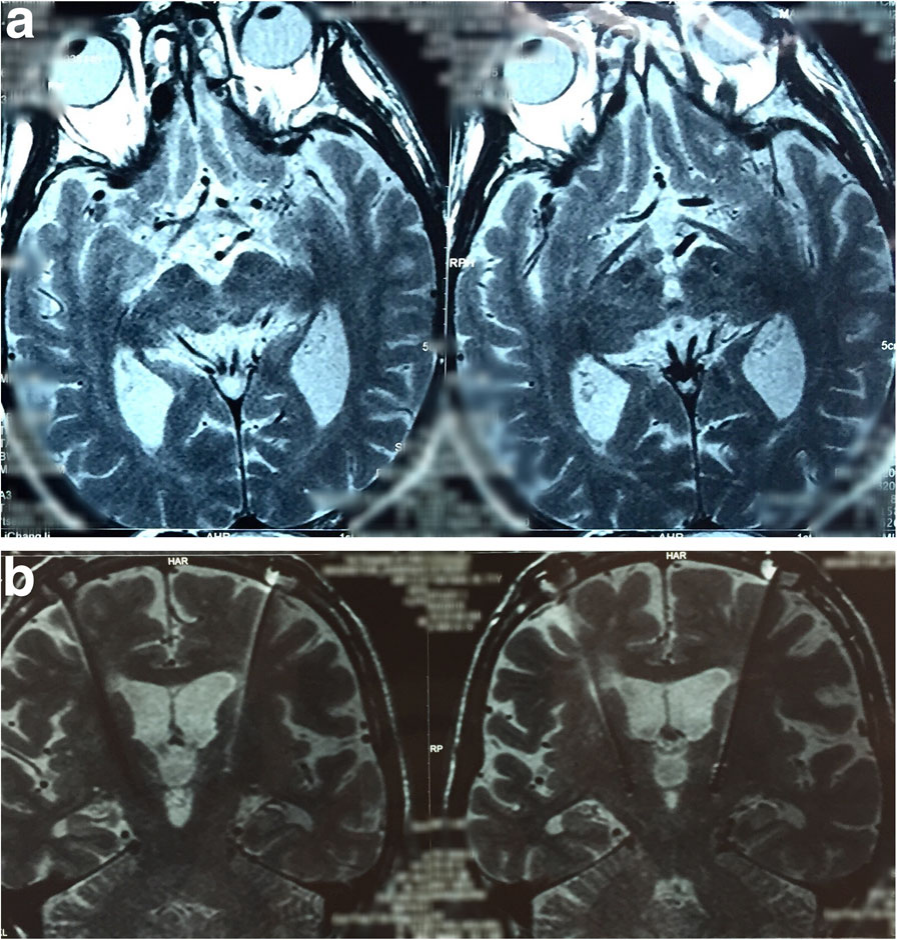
a. The lead location by the axial view of postoperative MRI. b. The lead location by the coronal view of postoperative MRI
